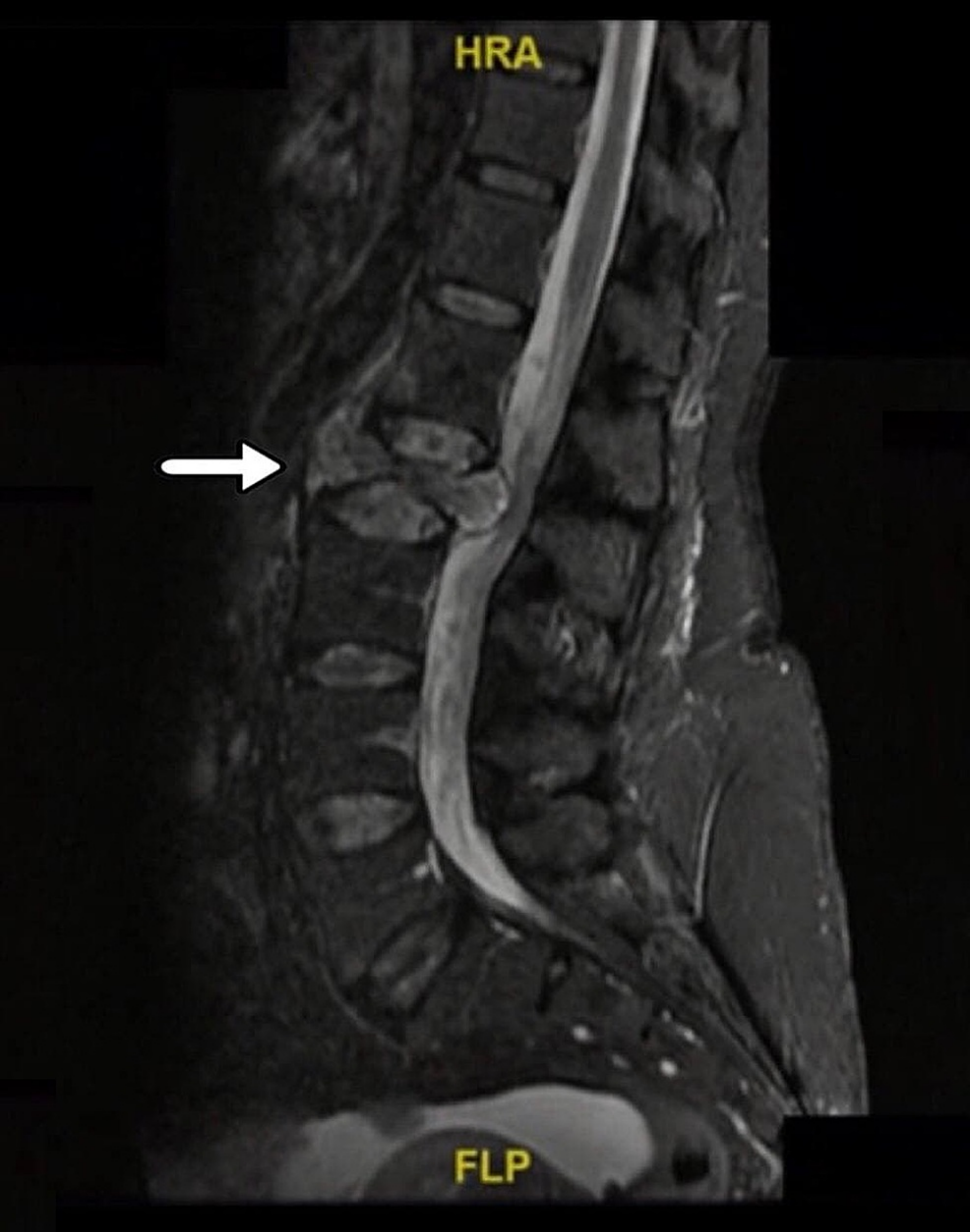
MRI scan lumbar spine Showing L2 compression fracture with significant height reduction
